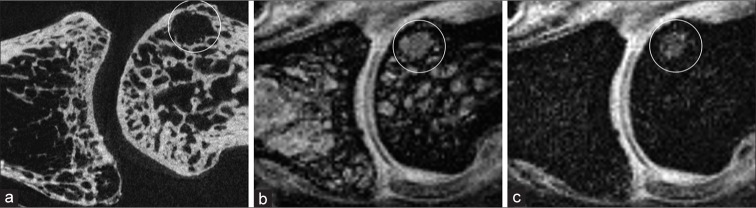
A subchondral defect (circle) was seen in this proximal interphalangeal joint on the sagittal (a) microcomputed tomography and (b) interleaved water-fat water + fat images. The (c) interleaved water-fat water-only image revealed its water content and identified it as a cyst.
